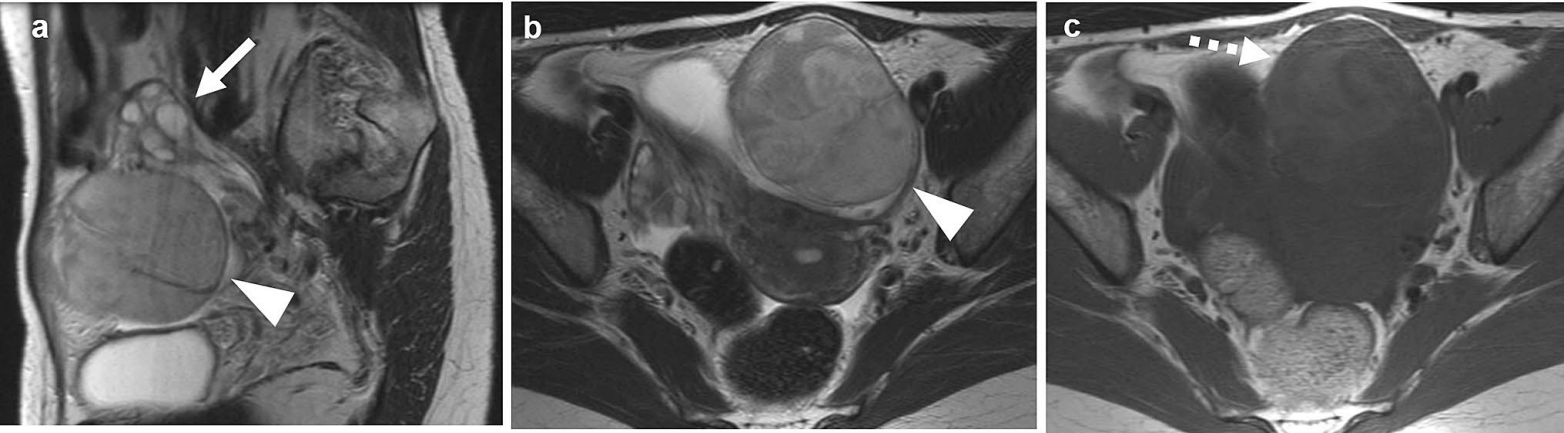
A 39-year-old woman presented with left lower abdominal pain of acute onset. a Sagittal T2-weighted image shows a tumor (arrowhead) located caudal to the left ovary (arrow). The tumor is enveloped by a thin layer of hypointensity, which is considered to be a fibrous capsule. b Axial T2-weighted MR image shows a well-defined solid tumor (arrowhead) of intermediate intensities ventral to the uterus. c Axial T1-weighted image shows areas of increased signals within the tumor (dashed arrow), suggestive of hemorrhage. Although torsion of the tumor was suspected by this finding, it was not apparent at surgery
